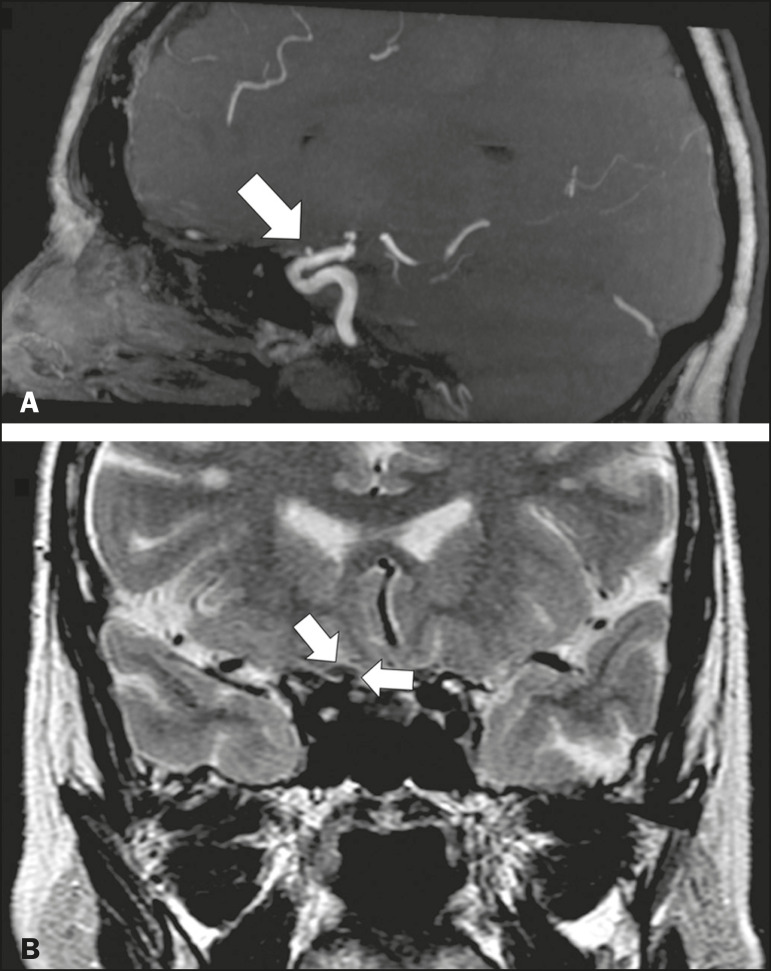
Ophthalmic artery aneurysm. A 65-year-old female with right temporal visual loss. Sagittal 3D time-of-flight magnetic resonance angiography (A) showing an aneurysm at the origin of the right ophthalmic artery (arrow). Coronal T2-weighted MRI scan (B) showing compression of the medial surface of the right optic nerve (oblique arrow) by the aneurysm (horizontal arrow).
